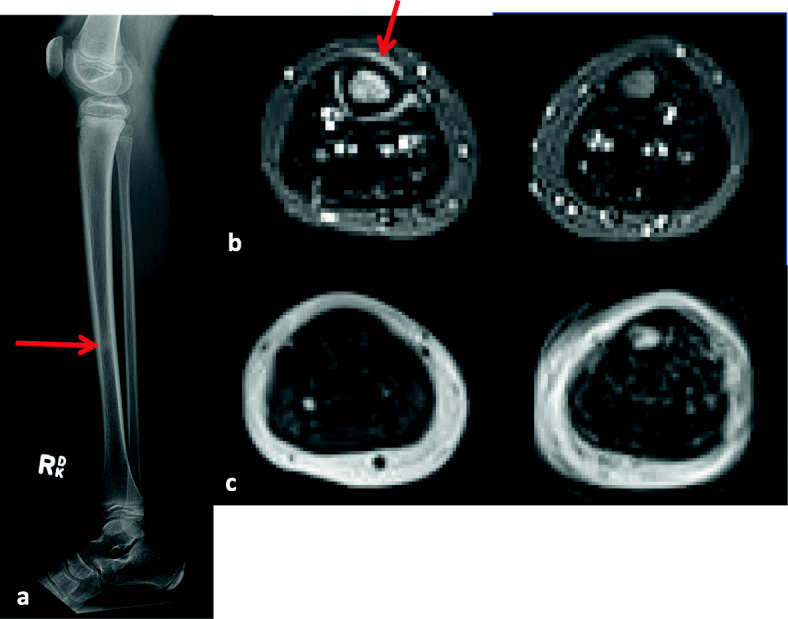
Case 6 (Initial observation) Lateral radiograph of the right lower extremity (a) shows a focal area of sclerosis in the mid tibial diaphysis with no associated periosteal or cortical thickening (arrow) Axial inversion recovery MR images of the thighs (b) obtained 2 weeks after the aforementioned x-rays show a focus of increased bone marrow signal in the mid right tibial diaphysis associated with a hyperintense periosteal halo on fluid sensitive images (arrow) which presents with low signal intensity on corresponding axial T1 images (c)
